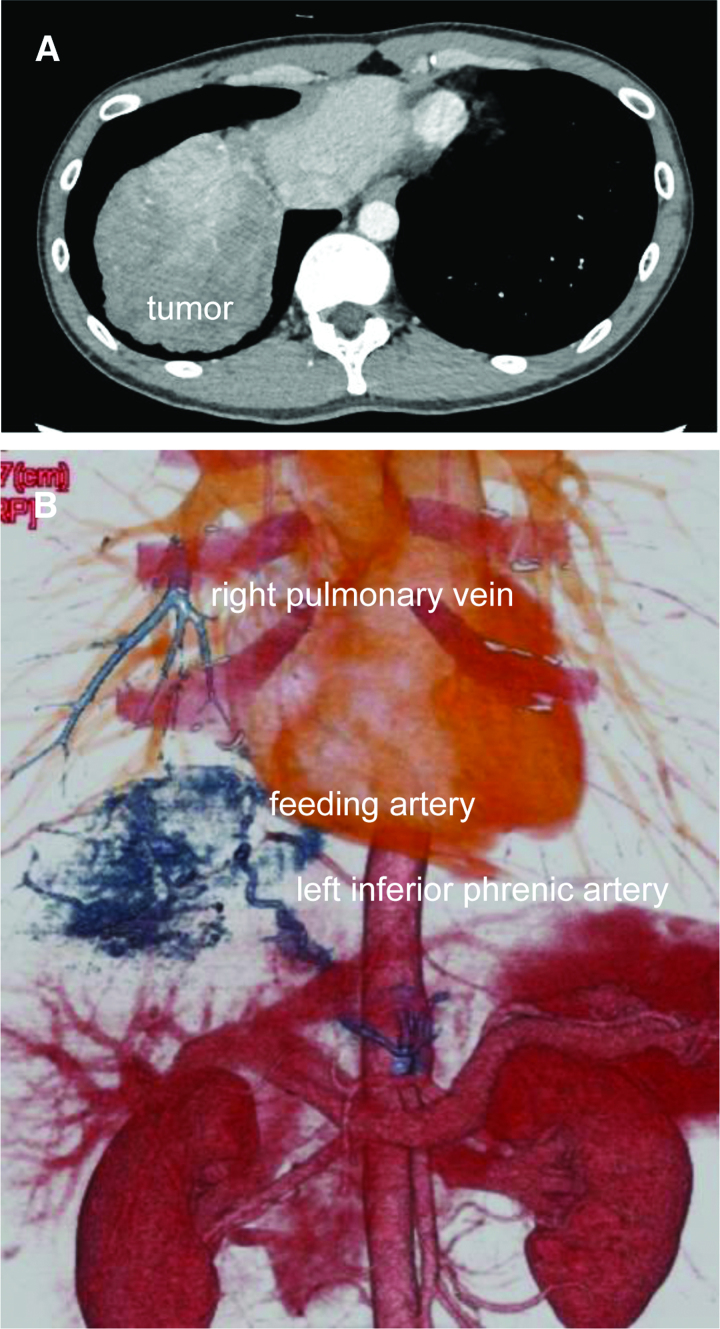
Chest computed tomography (CT) findings. (A) A tumorous lesion measuring 10 × 8 mm was identified in the right lung base. (B) The feeding artery to the tumor was found to branch off from the abdominal aorta. An anomalous vessel was observed branching from the abdominal aorta and sharing a common trunk with the left gastric artery, which in turn branched off from the anomalous vessel. The left inferior phrenic artery was found to branch off from the left gastric artery. The venous drainage from the tumor was flowing into the right pulmonary vein.
